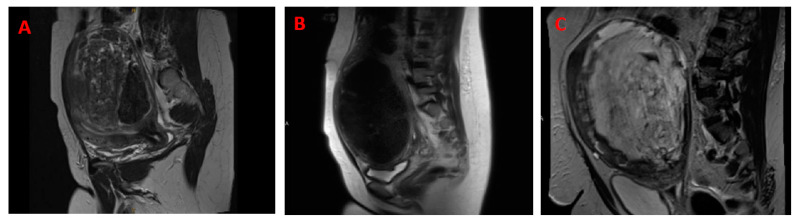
Reproductive-aged women with a large pelvic mass. Both presented with heavy menses, pelvic mass, and pressure. An MRI was obtained to better visualize large masses that were identified on the ultrasound. (A) A 45-year-old with a large pelvic mass. MRI: possible myxoid though features overlap with leiomyosarcoma. (B) A 44-year-old with a uterine mass. MRI: dominant anterior mass. Leiomyosarcoma cannot be entirely excluded. Pathology confirmed: benign leiomyoma. (C) A 38-year-old who presented with a pelvic mass and abnormal uterine bleeding; MRI sagittal view of a patient who presented with a pelvic mass and abnormal uterine bleeding.
